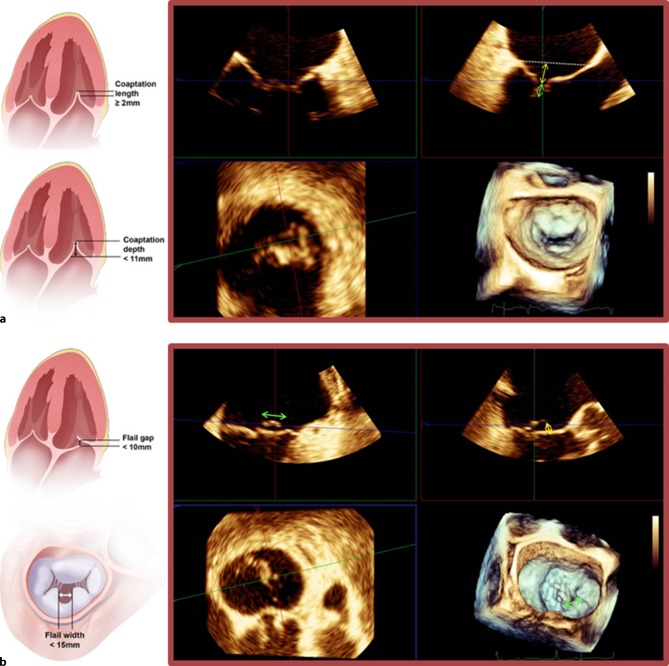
a–c Transoesophageal echocardiogram: assessment of mitral valve eligibility for MitraClip implantation
